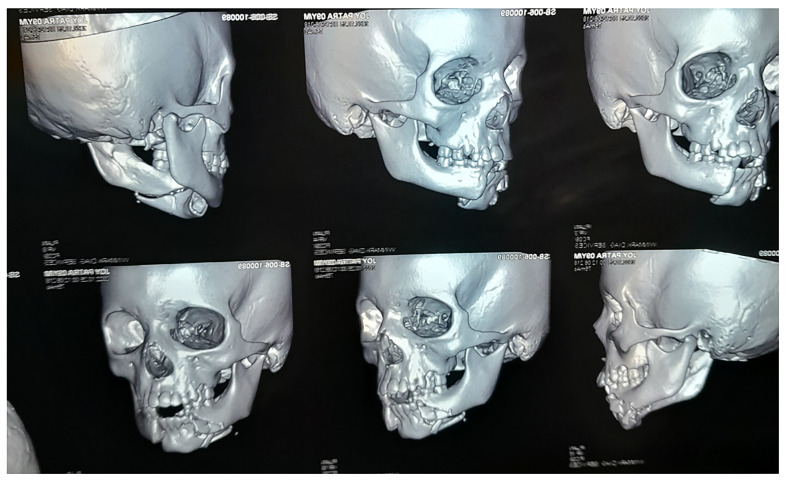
Cone beam computed tomographic image of a patient showing parasymphyseal fracture of mandible.
